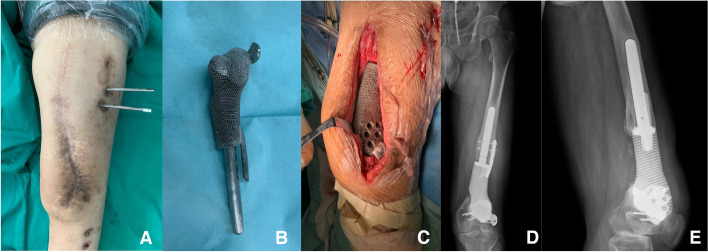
Anteroposterior and lateral radiographs at 36 months follow-up. A The wound healed well with no evidence of infection. B The metaphysis was considered insufficient to lock the intramedullary nail, an integrated intramedullary nail prosthesis was applied. C Prosthesis was stabilized using screws that penetrated the prosthesis. D Translucent zone surrounding the prothesis could be observed both on proximal and distal bone with no new bone growth. E Sclerosis of the distal interface
